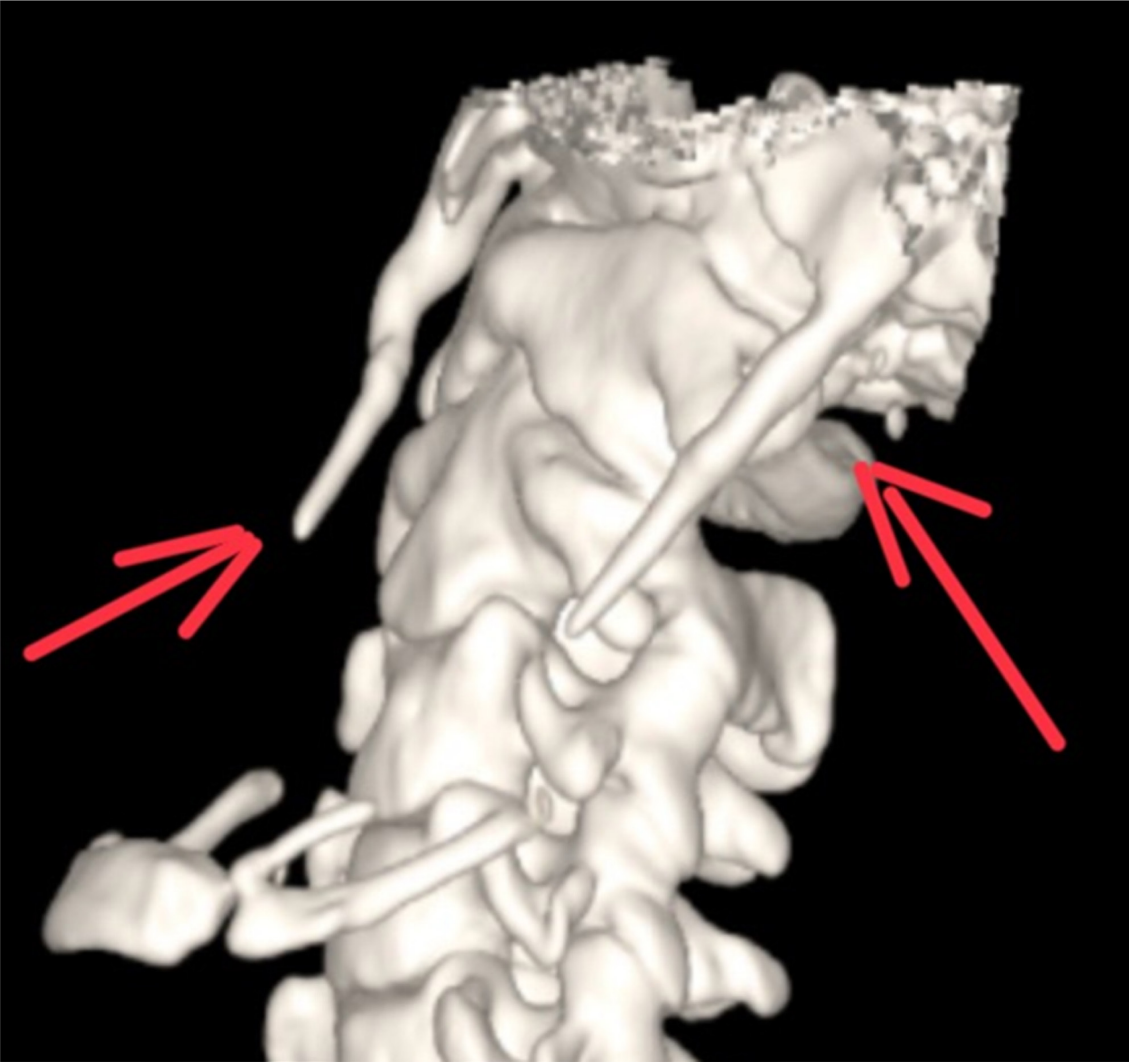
Three‐dimensional reconstructed CT image demonstrating bilateral elongated styloid processes projecting inferiorly, clearly visible on both sides of the skull. This finding supports the diagnosis of bilateral Eagle's syndrome.
